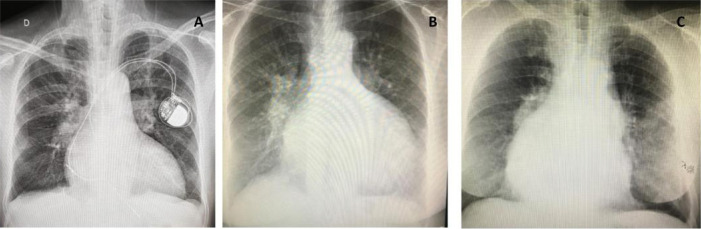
Chest radiographs of three patients with congenitally corrected
